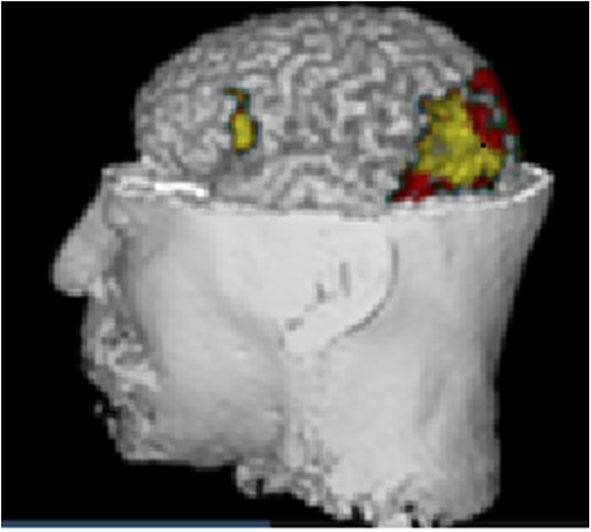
Morphometric analysis of the T1 MRI of the same case. Statistical parametric mapping using the plugin IBASPM based on the regional volume of the MRI in comparison with the normative data (Z values). The yellow area identified the regions with brain damage, occipital damage responsible of visual impairment, and lesion in Broca's area which explained the language impairment, not detected by MRI. The correspondence with Figure 4 is striking.
